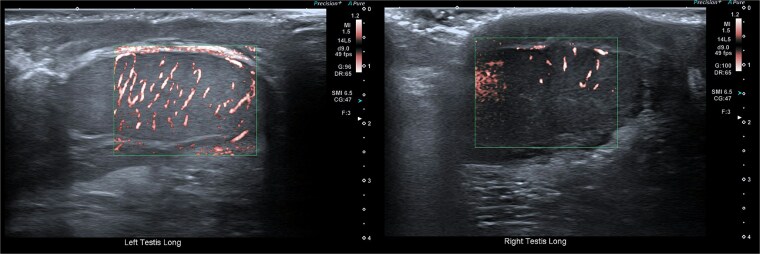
Follow-up ultrasonographic image at 4 weeks post-operatively, demonstrating the left testis within the scrotum, with restored homogeneous echotexture and normal intratesticular vascularity on colour Doppler.
